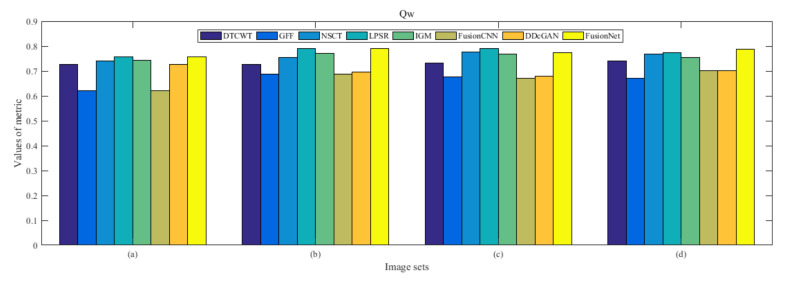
Values of $Q_{w}$ in the fused images (MRI-CBF): (a) is composed of eight images in Figure 42, (b) is composed of eight images in Figure 43, (c) is composed of eight images in Figure 44, (d) is composed of eight images in Figure 45.
